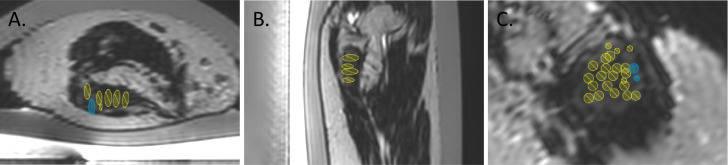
Representative T2-weighted treatment planning images in axial (A), sagittal (B) and coronal (C) views showing placements of MR-HIFU treatment cells (ellipsoids).
